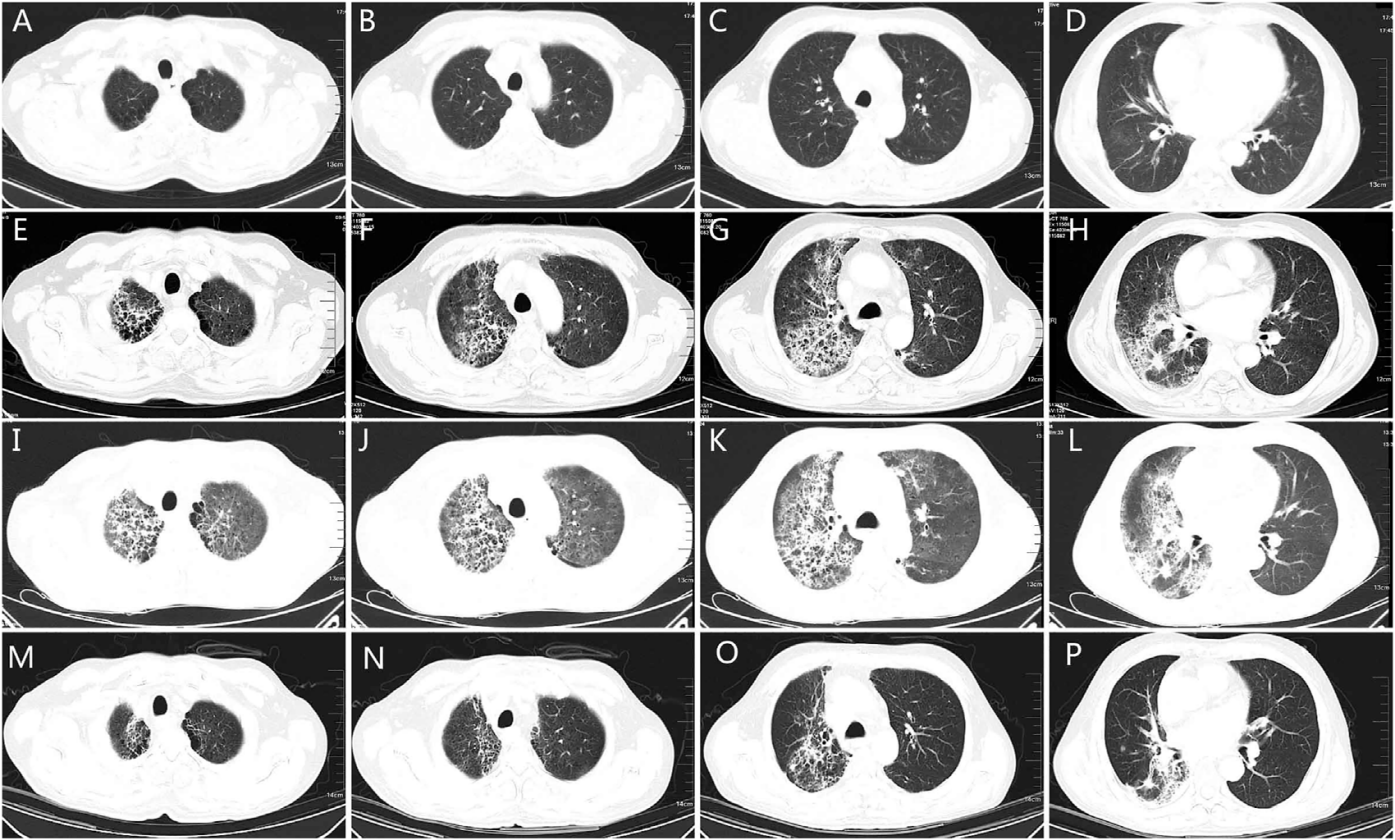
The chest CT of patient two. (A–D): chest CT showing the lung quality before afatinib. (E–H): chest CT showing pneumonia 27 days after afatinib therapy, the grid changes and ground glass hinting on lung injury. (I–L): chest CT 5 days after discontinuation of afatinib and empirical antibiotics, showing enlarged field of grid changes and pulmonary interstitial edema, indicating the diffused alveolar injury. (M–P): chest CT 17 days after glucocorticoid therapy, the grid changes and pulmonary interstitial edema reduced significantly, indicating improvements of lung injury. CT: computed tomography.
